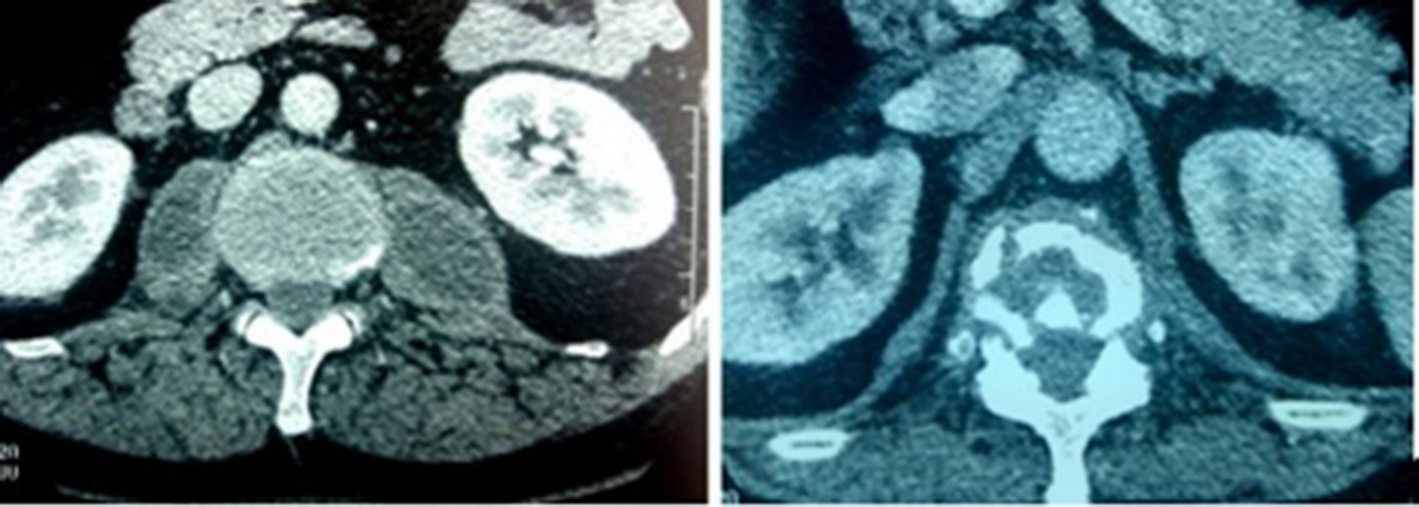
Abscess of both psoas with spondylodiscitis on a cross -section (CT).
